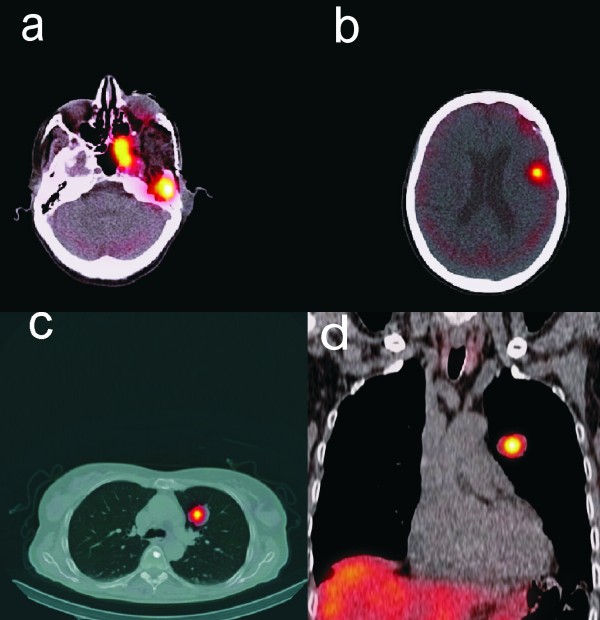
SPECT/CT images of somatostatin receptor scintigraphy display avid uptake in the intracranial meningioma (2a and b) as well as in the pulmonary metastases (2c and d).
